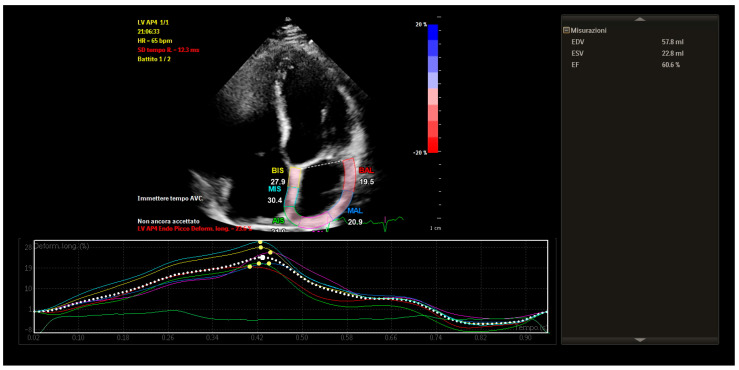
Speckle—tracking echocardiography showing mildly reduced atrial strain with preserved mechanical synchrony.
